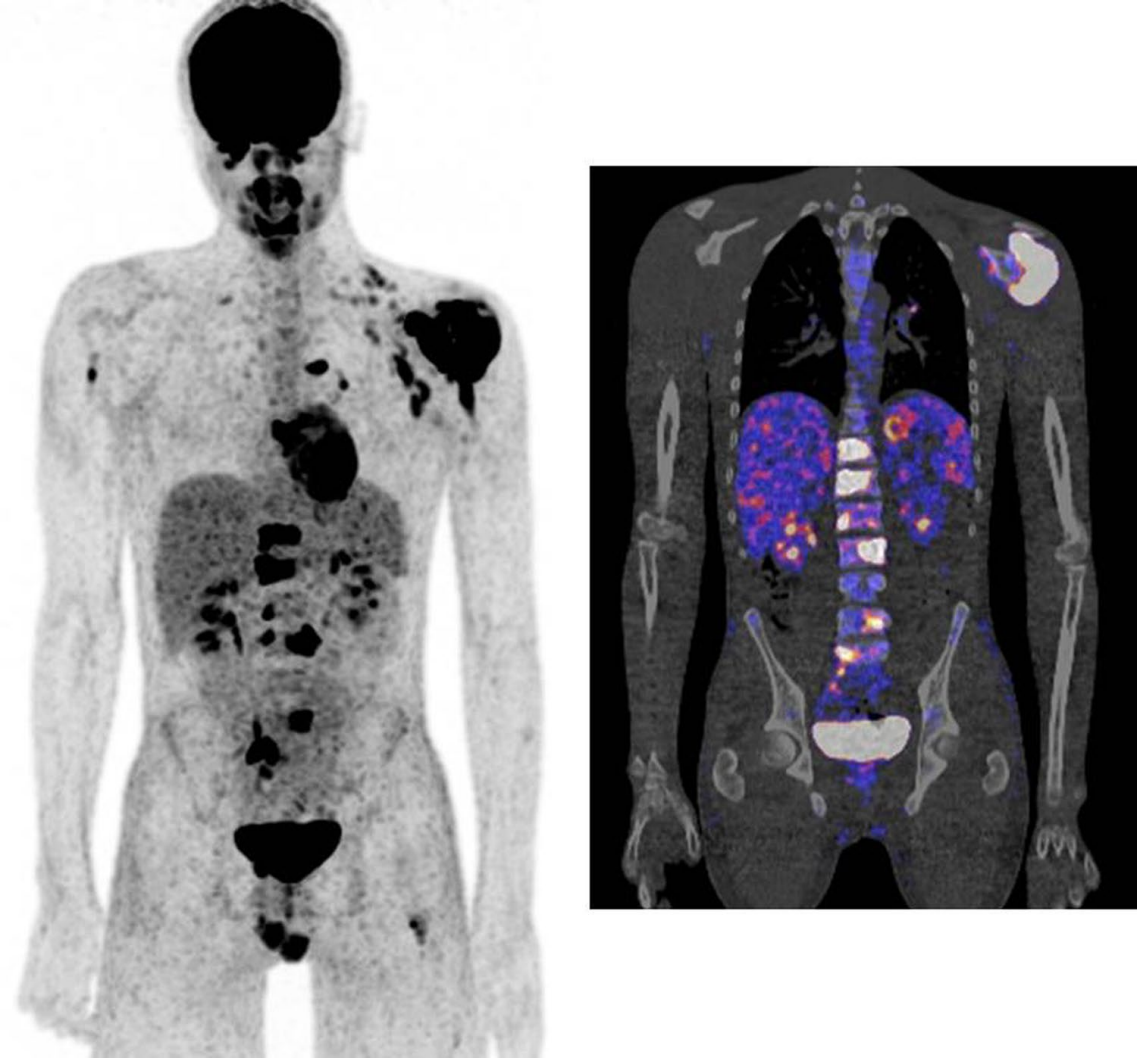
Patient with a proven tuberculosis osteomyelitis of the left shoulder. FDG-PET was performed to identify any disseminated foci of infection. FDG-PET image (*left*) and fusion PET-CT image (*right*) showing multiple infectious foci in the body (left shoulder, multiple vertebras, right upper arm, left upper leg)
